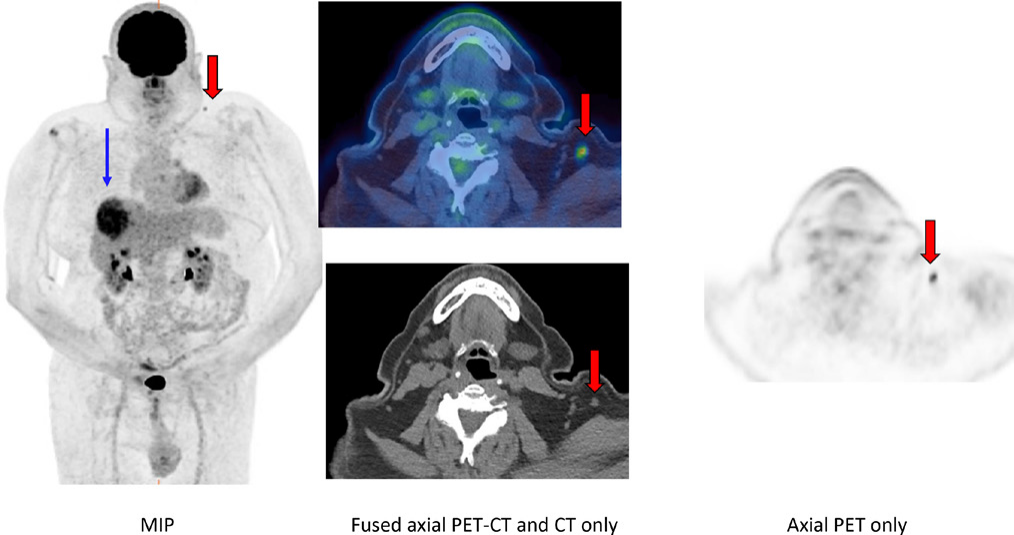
FDG PET-CT (MIP, axial PET only, axial fused and axial CT only) revealed asymmetrical, intense uptake in left supraclavicular normal-sized lymph node (red arrows). The lymph node demonstrated a normal fatty hilum. No asymmetrical uptake was seen in deltoid musculature. MIP images demonstrate intense uptake in primary liver lesion (blue arrow).
